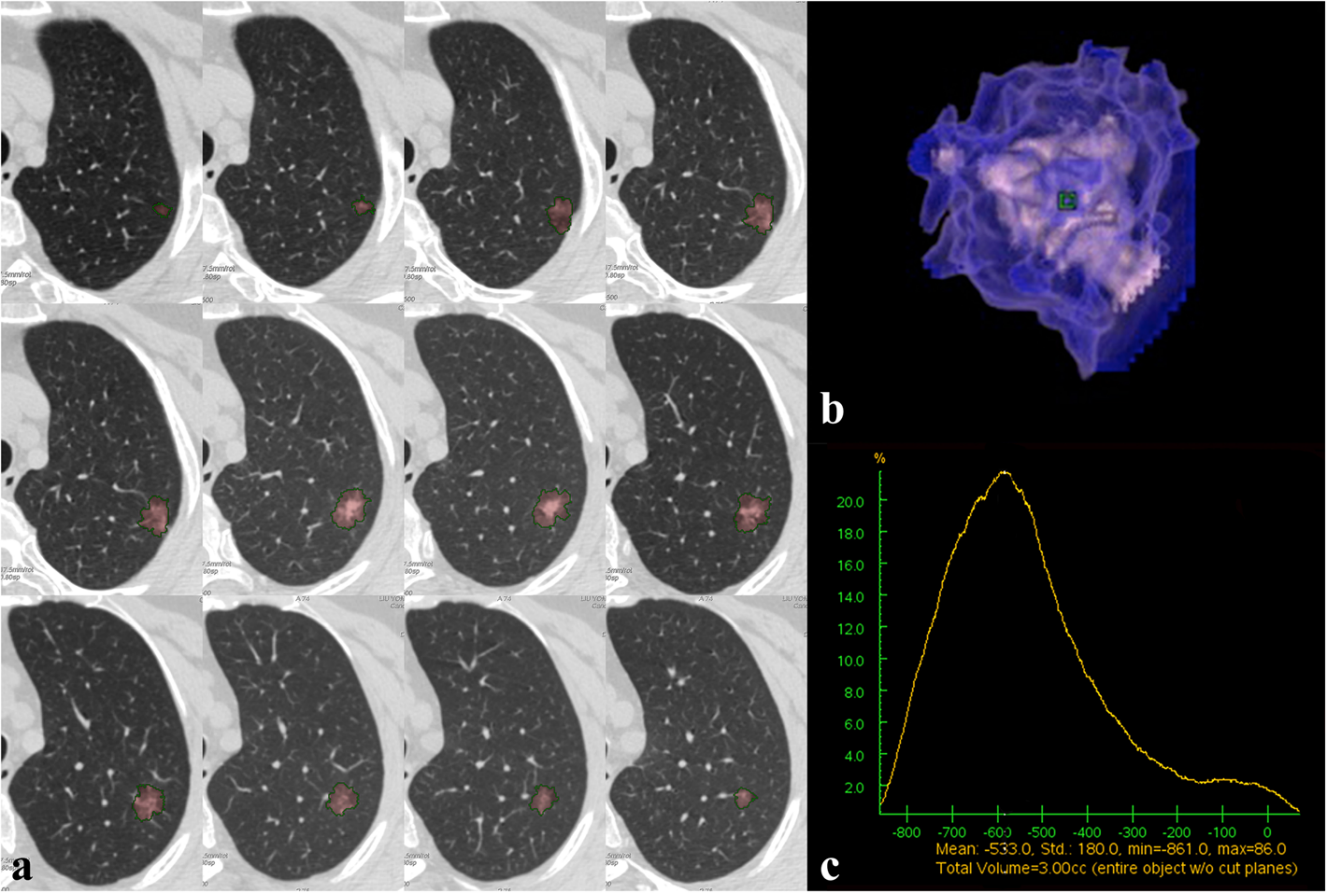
Mean CT value calculated using three-dimensional (3D) computer-quantified CT number analysis. a CT images show computer-generated tumor boundaries that were inspected and edited slice-by-slice by radiologist for correctness and consistency. b 3D image of the tumor generated automatically by the computer. c CT number distribution curve of the tumor. The mean CT value of this tumor is − 533 HU ranging from − 861 HU to 86 HU
